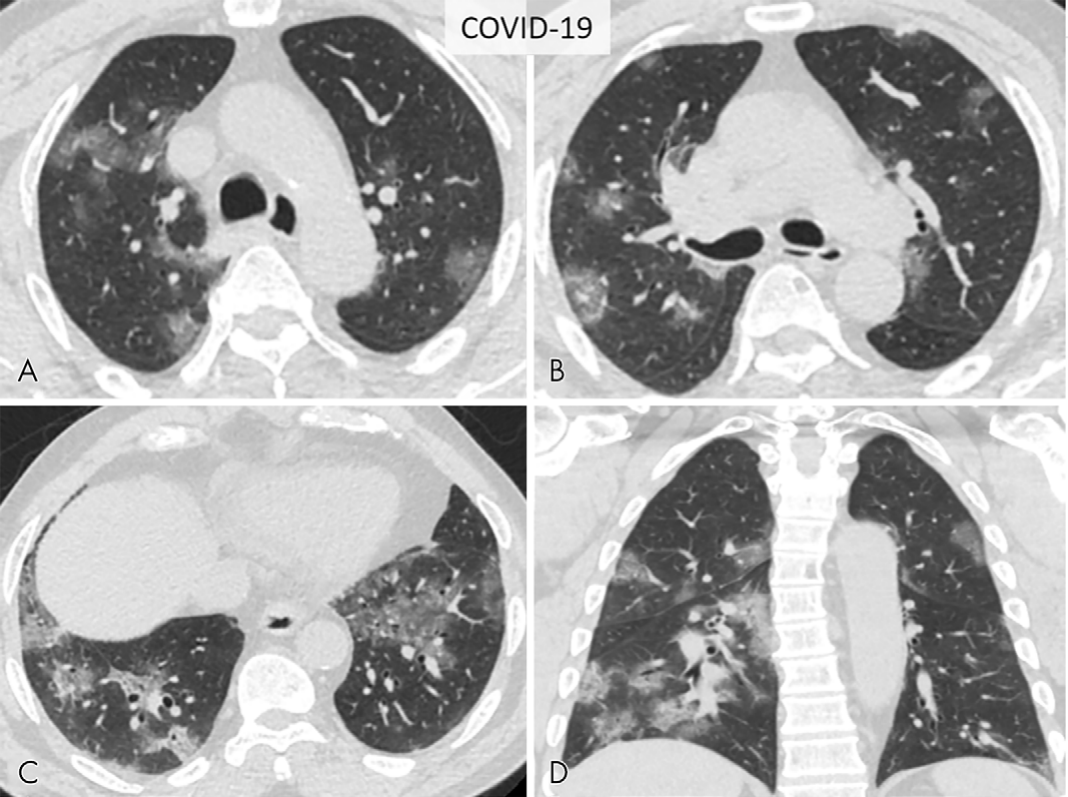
Typical CT imaging features for COVID-19. Unenhanced thin-section axial (*A–C*) and coronal multiplanar reformatted images (*D*) of the lungs in a 77-year-old man with a positive RT-PCR show bilateral, multifocal rounded and peripheral GGO. Routine screening CT for diagnosis or exclusion of COVID-19 is currently not recommended by most professional organizations or the U.S. Centers for Disease Control and Prevention.
